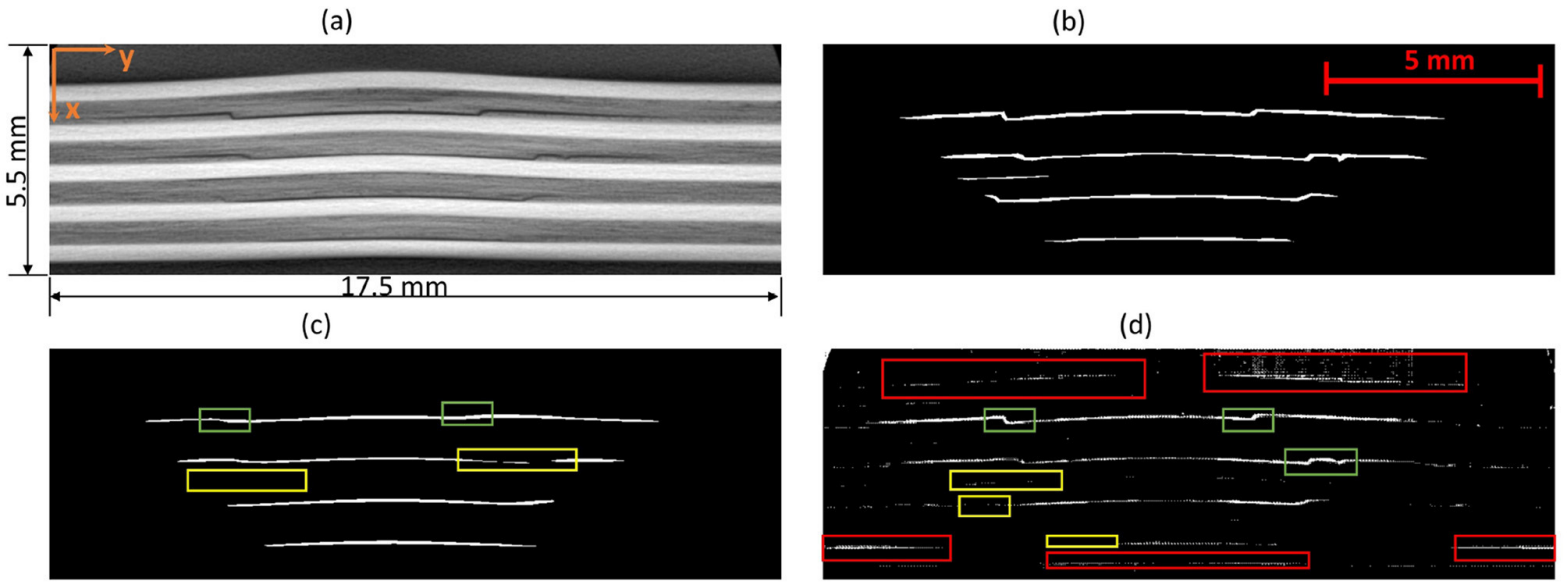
An example CT slice of 7.5J impact energy with damage masks. Red boxes show false positives by SAM, yellow boxes show false negatives by both models, and green boxes highlight SAM's superior performance over Detectron2 in learning complex damage features. (a) CT slice for 7.5J. (b) Mask-Hand-annotation. (c) Mask-Detectron2. (d) Mask-SAM.
